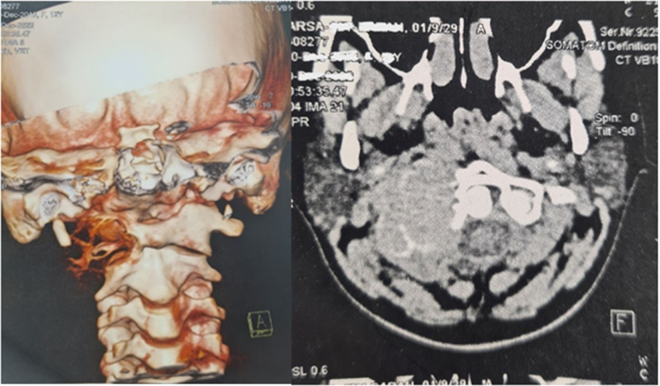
The cervical CT scan without contrast shows a 30 × 36 × 49 mm lytic-expansile mass lesion with bony erosion in the right transverse process of Atlas. Left side: axial view; right: 3D reconstruction.
